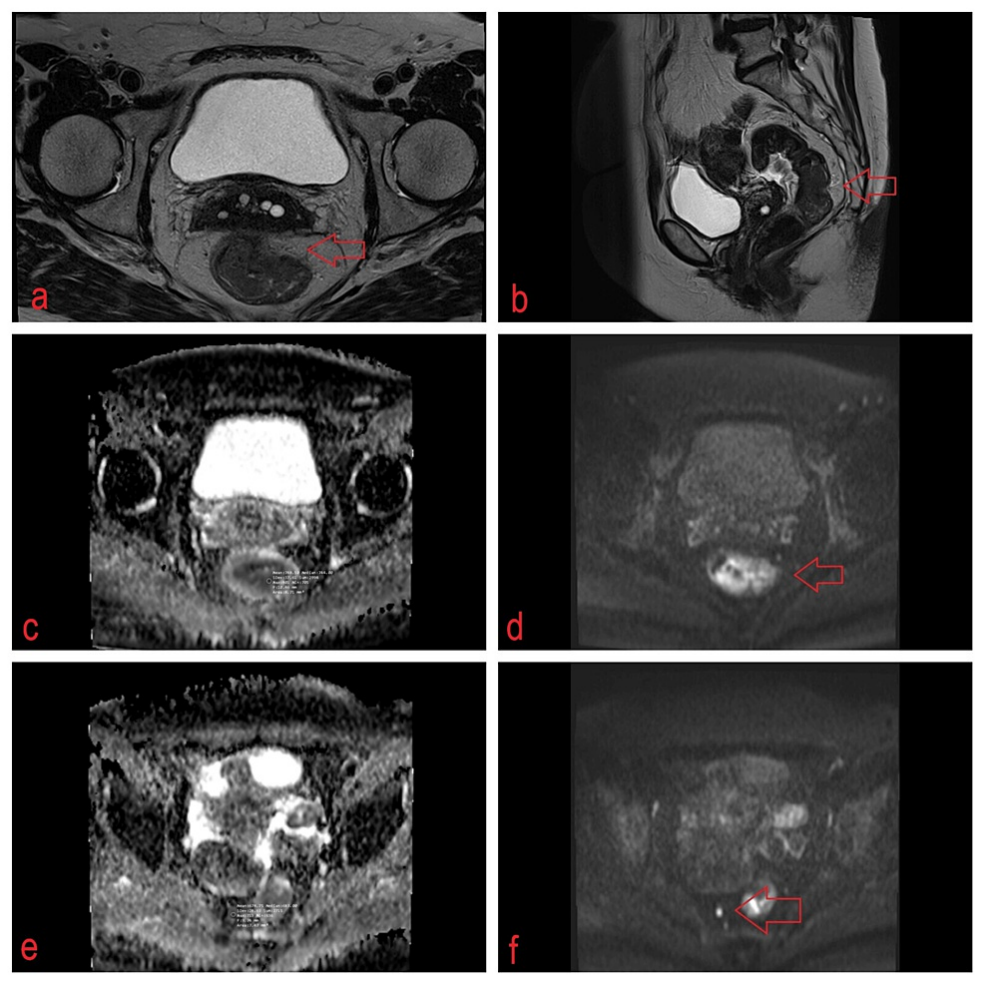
Rectal cancer staged as T3bN2 involves mesorectal fascia and is associated with multiple enlarged mesorectal lymph nodes. Rectal cancer ADC value was 0.748 * 10^−3^ mm^2^/s; suspicious lymph node ADC value was 0.678 * 10^−3 ^mm^2^/s. (a) Axial T2; (b) Sag T2; (c) rectal cancer ADC map and (e) lymph node ADC map; (d) rectal cancer high-b value (b = 1000 s/mm^2^) DWI image and (f) lymph node high-b value (b=1000 s/mm^2^) DWI image. ADC: apparent diffusion coefficient; DWI: diffusion-weighted imaging.
